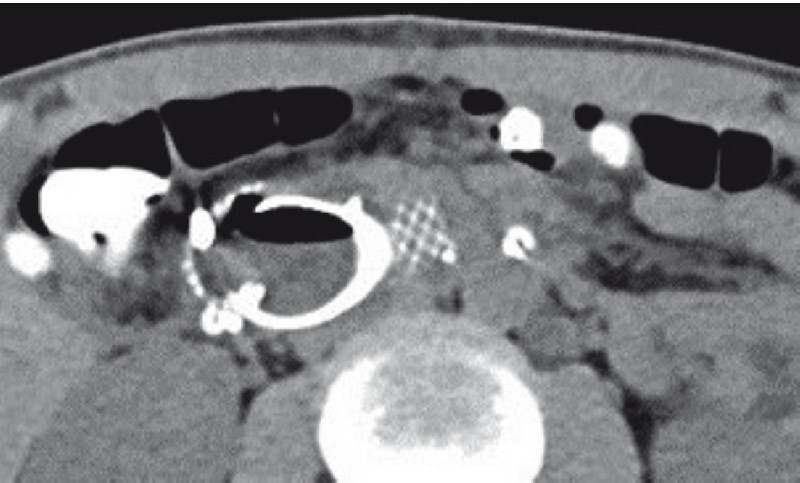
Follow-up computed tomography scan 15 days after the procedure showing no retroperitoneal or intraperitoneal fluid collections, with the self-expandable metal duodenal stent remaining in position.
